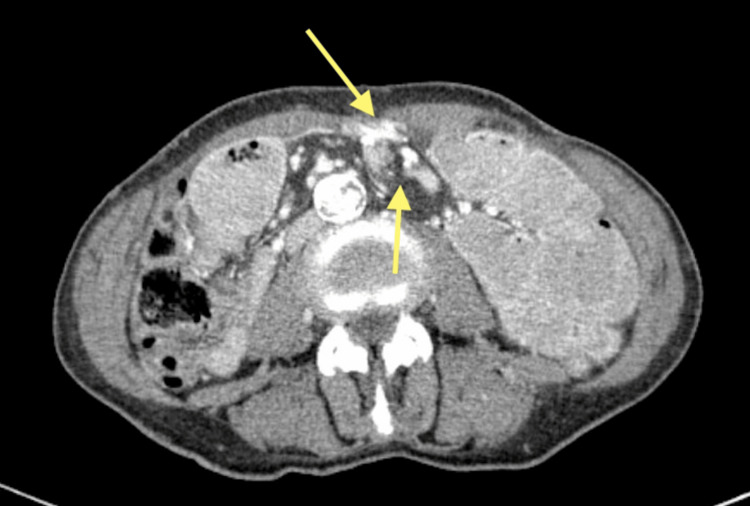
CT scan showing the transition point with intestinal rotation and a mesenteric vessel swirl. Proximal intestinal distention is seen due to rotation, accompanied by a mesenteric vessel swirl (yellow arrows). Beyond the transition point, intestinal loops are either collapsed or of normal caliber.
